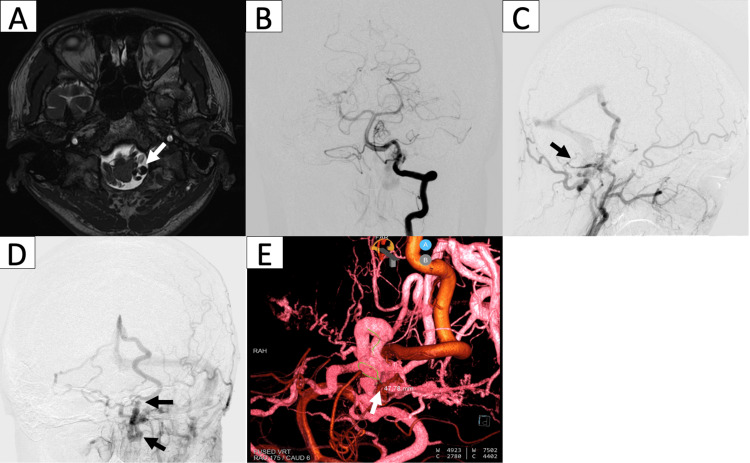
Preoperative imaging MRI shows a flow void (white arrow) on the T2-weighted image at the level of the foramen magnum (a). DSA with vertebral artery (b) and external carotid artery (c) injections show fistula with feeding arteries from branches of the ascending pharyngeal artery (white arrowhead), occipital artery (black arrow), and vertebral artery (white arrow). Venous phase (d) shows a draining vein that continues into the basal vein of Rosenthal (black arrow) and descending posterior spinal vein (black arrowhead). 3D DSA of both external and vertebral arteries depict the shunt point (white arrow) and feeder arteries (white arrowheads) (e). DSA: digital subtraction angiography
